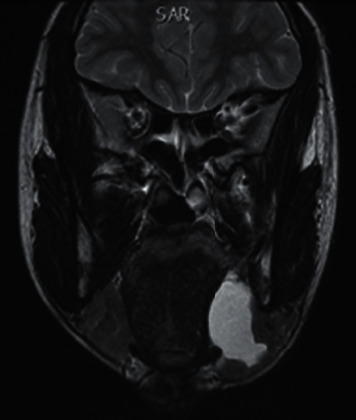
Magnetic resonance imaging T2 sequence, the cystic process of 31 × 31 × 17 mm occupying the lingual pelvis.
